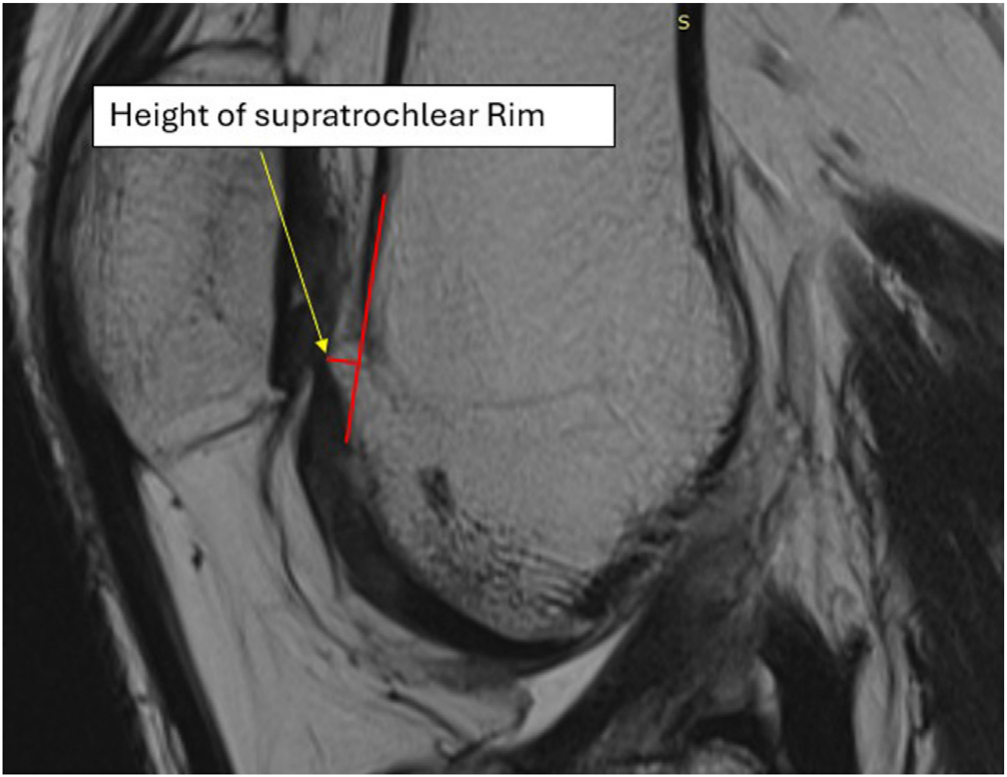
Magnetic resonance imaging sagittal slice showing measurement of height of supratrochlear rim. The measurement is taken on magnetic resonance imaging in the sagittal view, in which the deepest point of the trochlear groove is visible. The rim height (short red line) is measured from the extension of the frontal femoral cortex (long red line) to the most prominent anterior point of the supratrochlear rim.
